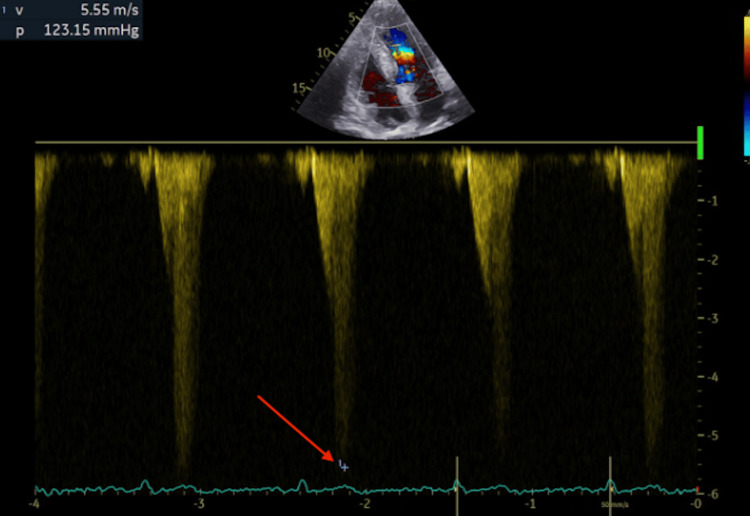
Continuous-Wave Doppler Assessment of Dynamic LVOT Obstruction Continuous-wave Doppler aligned with the aliasing jet demonstrates a late-peaking dagger-shaped systolic envelope consistent with dynamic LVOT obstruction, with a peak velocity of 5.55 m/s and an estimated peak gradient of 123 mmHg (red arrow)
